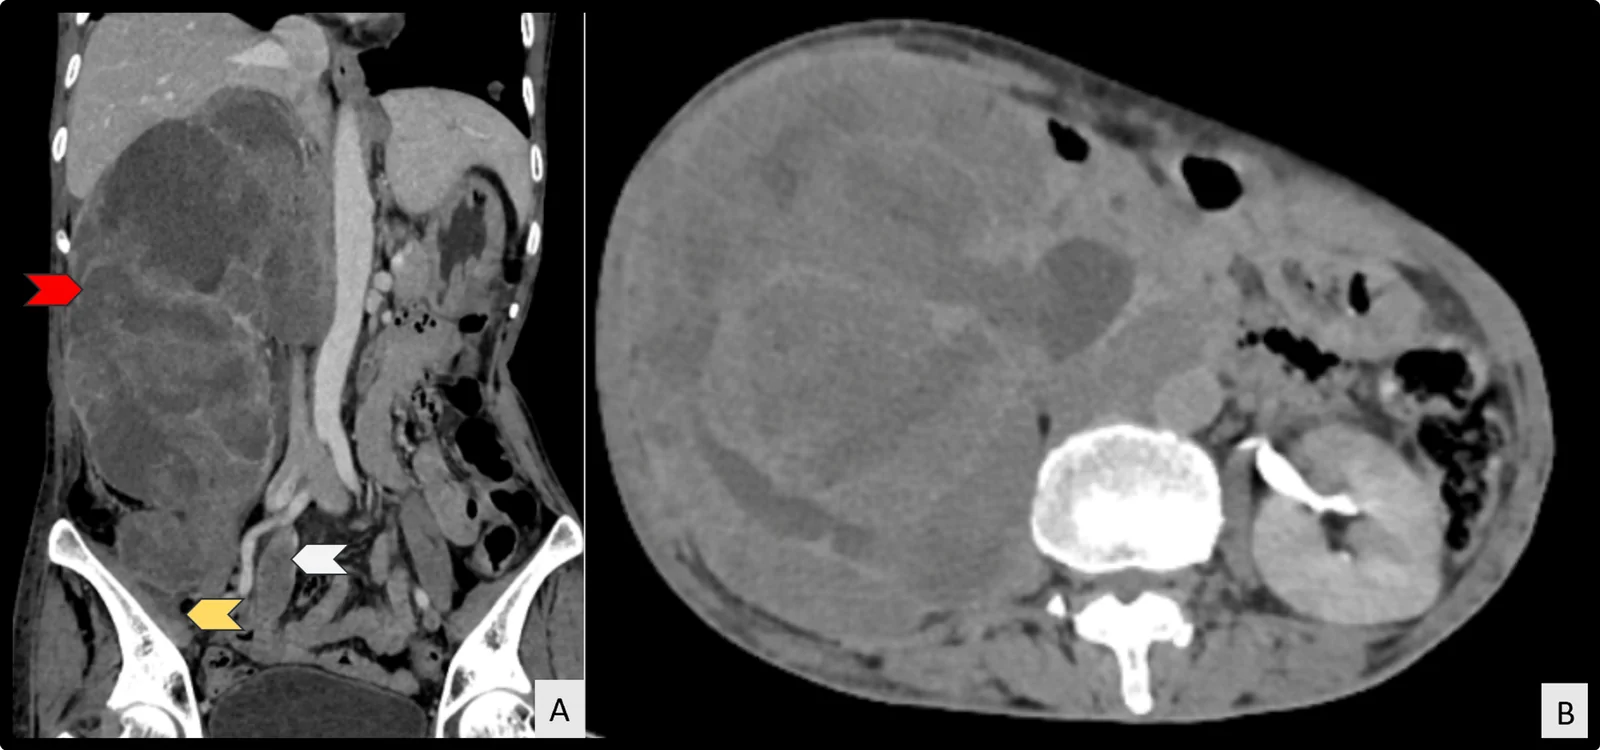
CECT of the reniform morphology and renal function A: Coronal reconstructed corticomedullary phase of the CT urography image demonstrates preservation of the reniform (bean-shaped) configuration of the enlarged right kidney despite near-complete replacement by the tumor (red arrowhead). The lesion shows extension into the lower ureter (white arrowhead) with focal infiltration into the right iliacus muscle (yellow arrowhead) B: Axial delayed excretory-phase CT urography image demonstrates absence of contrast excretion from the right kidney, indicating loss of renal function secondary to extensive tumor involvement. CECT: Contrast-enhanced computed tomography
